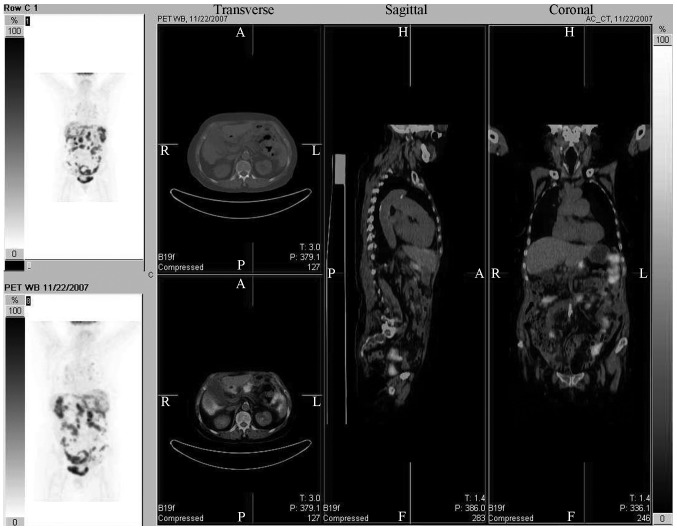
Positron emission tomography and computed tomography scans showed widespread metastases in the body of the patient on November 22, 2007.
